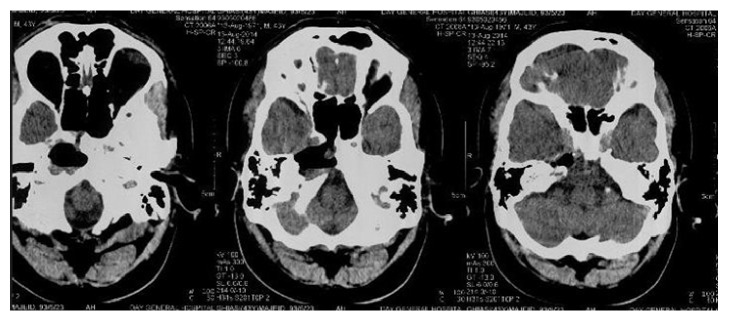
Axial CT scan shows acceptable aeration of right sphenoid sinus and previous location of the cyst.
